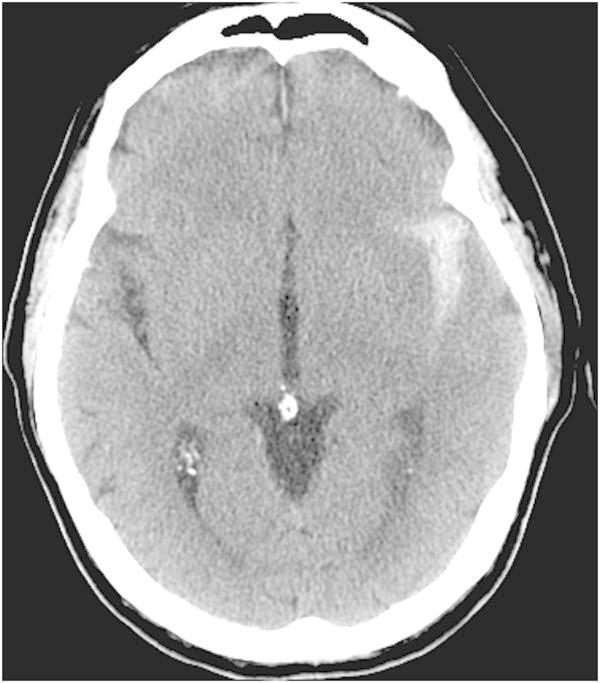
Initial CT-Head reveals SAH concentrated in the left sylvian fissure with a thickness of > 1.0 mm (Fisher Grade 3).
